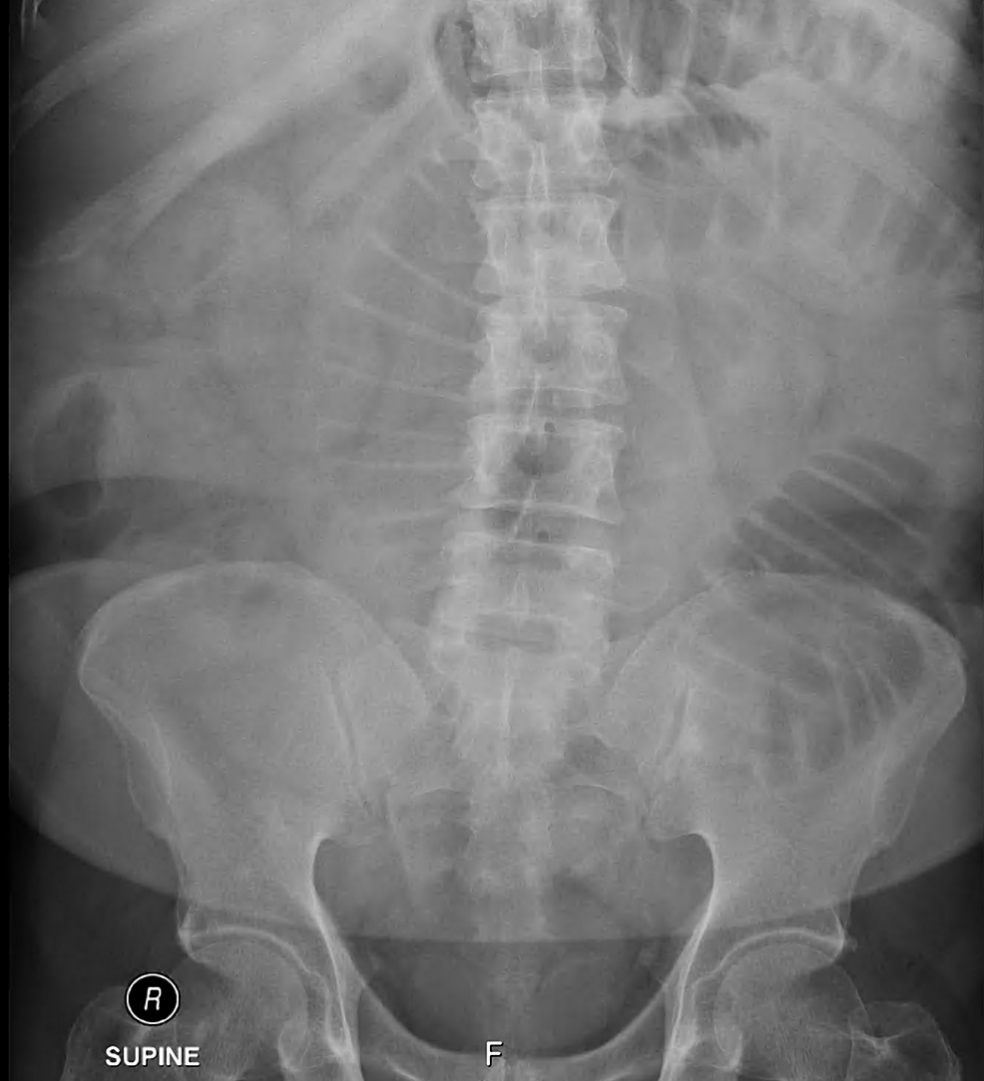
Plain abdominal radiograph showing multiple air-fluid levels indicating small bowel obstruction
